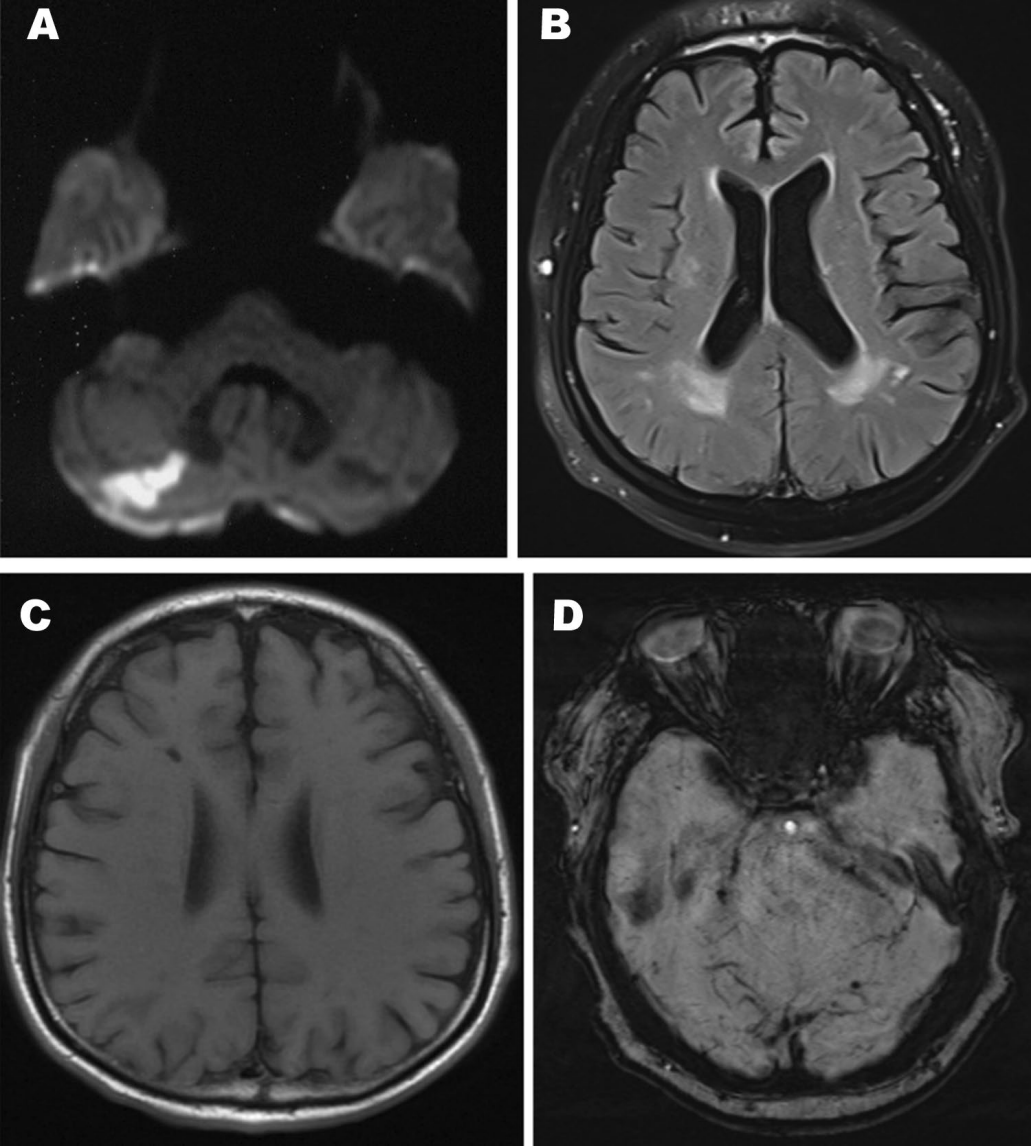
The brain MRI changes in Fabry disease. A DWI sequence showed recent cerebral infarctions in cerebellum. B T2 flair sequence showed white matter hyperintensity in parietal-occipital lobe and basal ganglia. C T1 sequence showed lacunar infarction in basal ganglia. D SWI sequence showed cerebromicrobleeds in infratentorial areas
